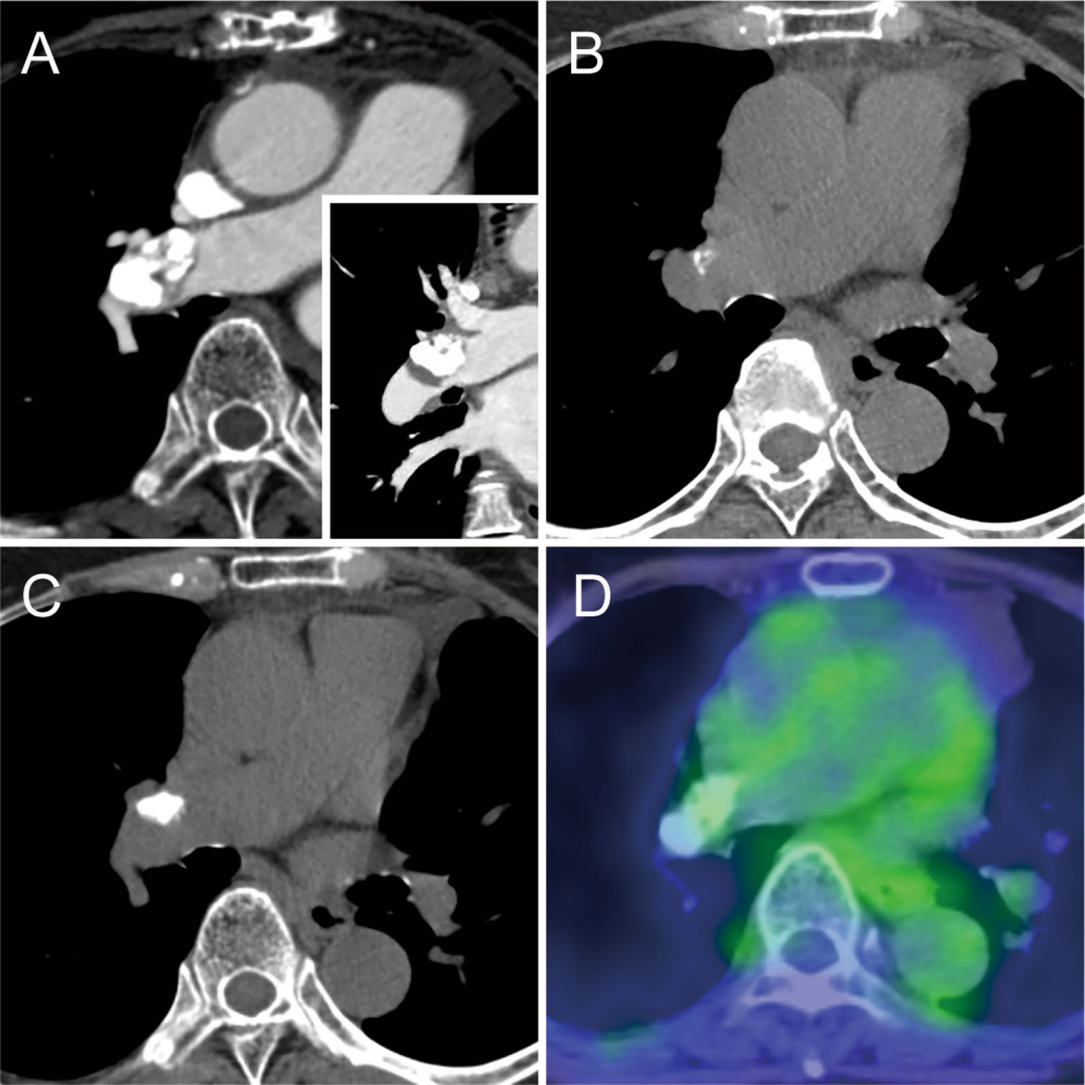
A Enhanced computed tomography (CT) showing a calcified nodule in the right pulmonary artery. Inset showing reconstructed frontal section. B CT performed 2 years before the first visit to our department showing a smaller calcified lesion. C CT performed 1 year before the first visit to our department showing an enlargement of lesion that was not recognized at this time. D The fluorodeoxyglucose (FDG) positron emission tomography CT showing abnormal accumulation of FDG in the lesion with a standardized uptake value max of 2.16
